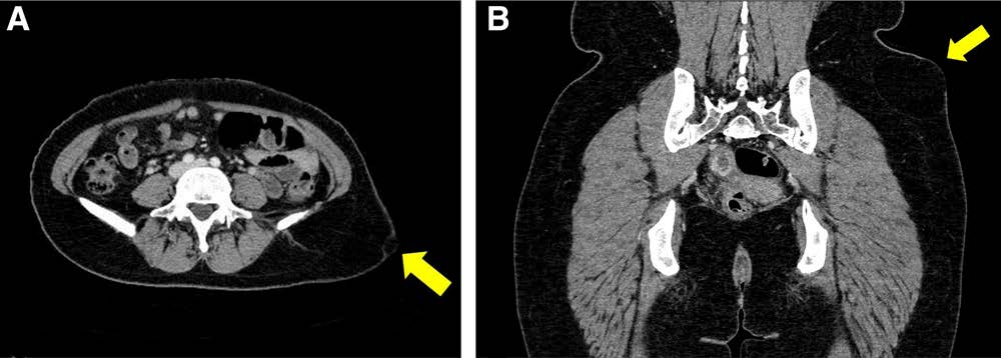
Computed tomography scan revealing a 5.7 × 8.4 × 5.8 cm fatty mass (yellow arrow) in the subcutaneous layer of the left lower flank. (A) Cross-sectional view and (B) coronal view.
